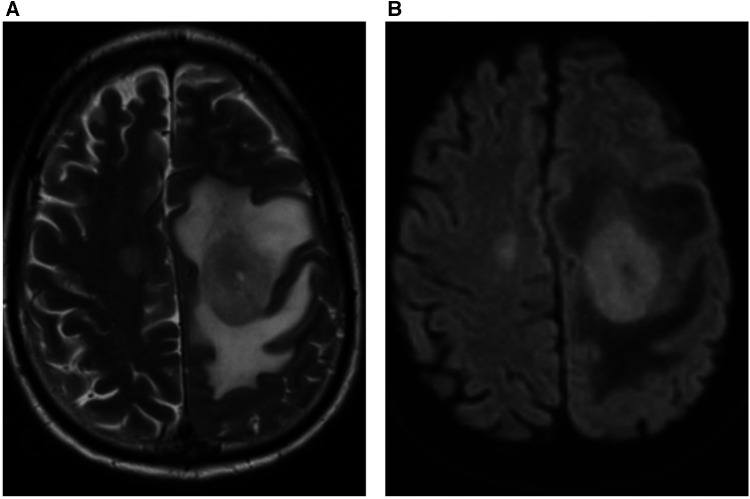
59 year-old male patient presented with right arm and leg weakness. (A) Axial T2-weighted MRI head sequence demonstrates an ovoid lesion in the left centrum semiovale which is hyperintense relative to grey matter. There are features consistent with surrounding peri-lesional vasogenic oedema. There is a further smaller lesion in the right centrum semiovale. (B) Both lesions demonstrate homogenous, increased diffusion restriction relative to background brain parenchyma on the axial DWI MRI head sequence. The patient was diagnosed with primary cerebral lymphoma based on imaging features and cerebrospinal fluid (CSF) analysis.
